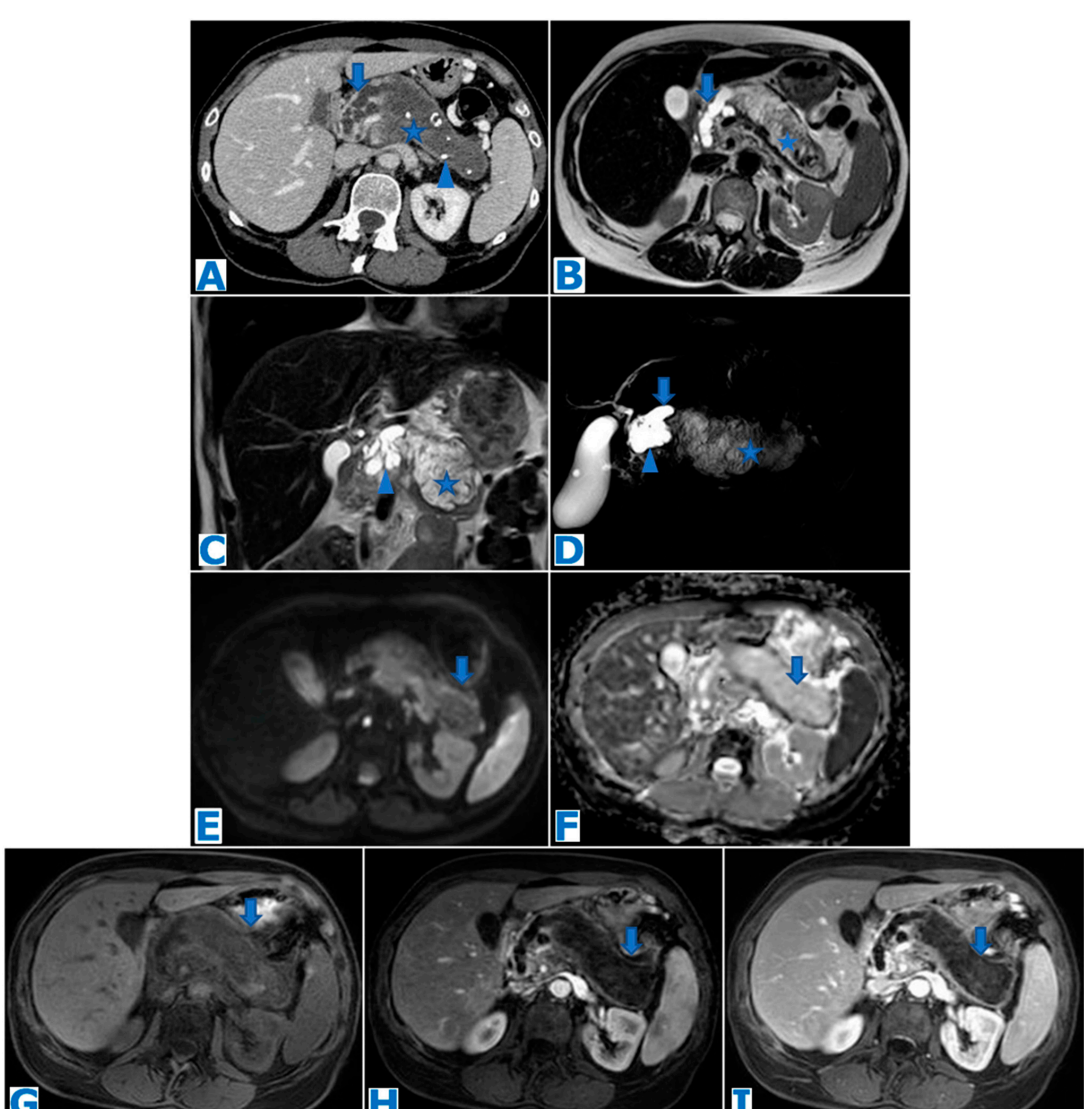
(A): axial CE portal venous phase CT, (B): axial T2WI, (C): coronal T2WI, (D)*:* MRCP, (E): DWI, (F): ADC, (G): axial NCE FST1WI, (H): CE pancreatic parenchymal phase FST1WI, (I): axial CE portal venous phase FST1WI. Colloid carcinoma. Patient is a 52-year-old woman with left upper quadrant pain for the previous six months and weight loss (15 kg). Blood laboratory tests are anodyne. Observe the intraluminal mass within the body and distal pancreas (* in (A–D)), notice its salt and pepper pattern in T2WIs (* in (B,C)) and how it is partly calcified (arrowhead in (A)). The lesion is associated with downstream MPD dilatation (arrow in (A,B,D)) and side branch ecstasy (arrowhead in (C,D)). There is no diffusion restriction (arrow in (E,F)). In the dynamic sequences following intravenous contrast administration, the tumour shows gradual enhancement of the periphery and the subtle septa (arrows in (G–I)). Patient underwent a cephalic duodenopancreatectomy and diagnosis was pathologically proven.
